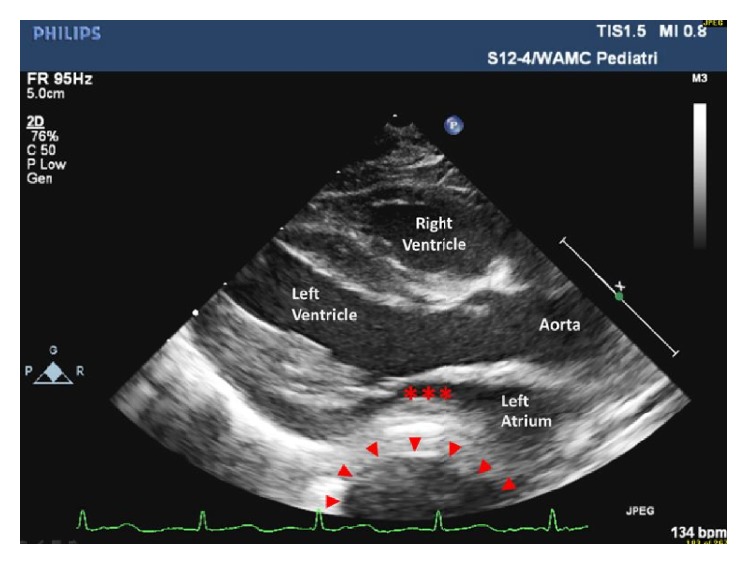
Parasternal long axis showing anterior deviation of the left atrium with anatomical impingement of the mitral valve annulus (*∗*) by the stomach (arrowhead).
